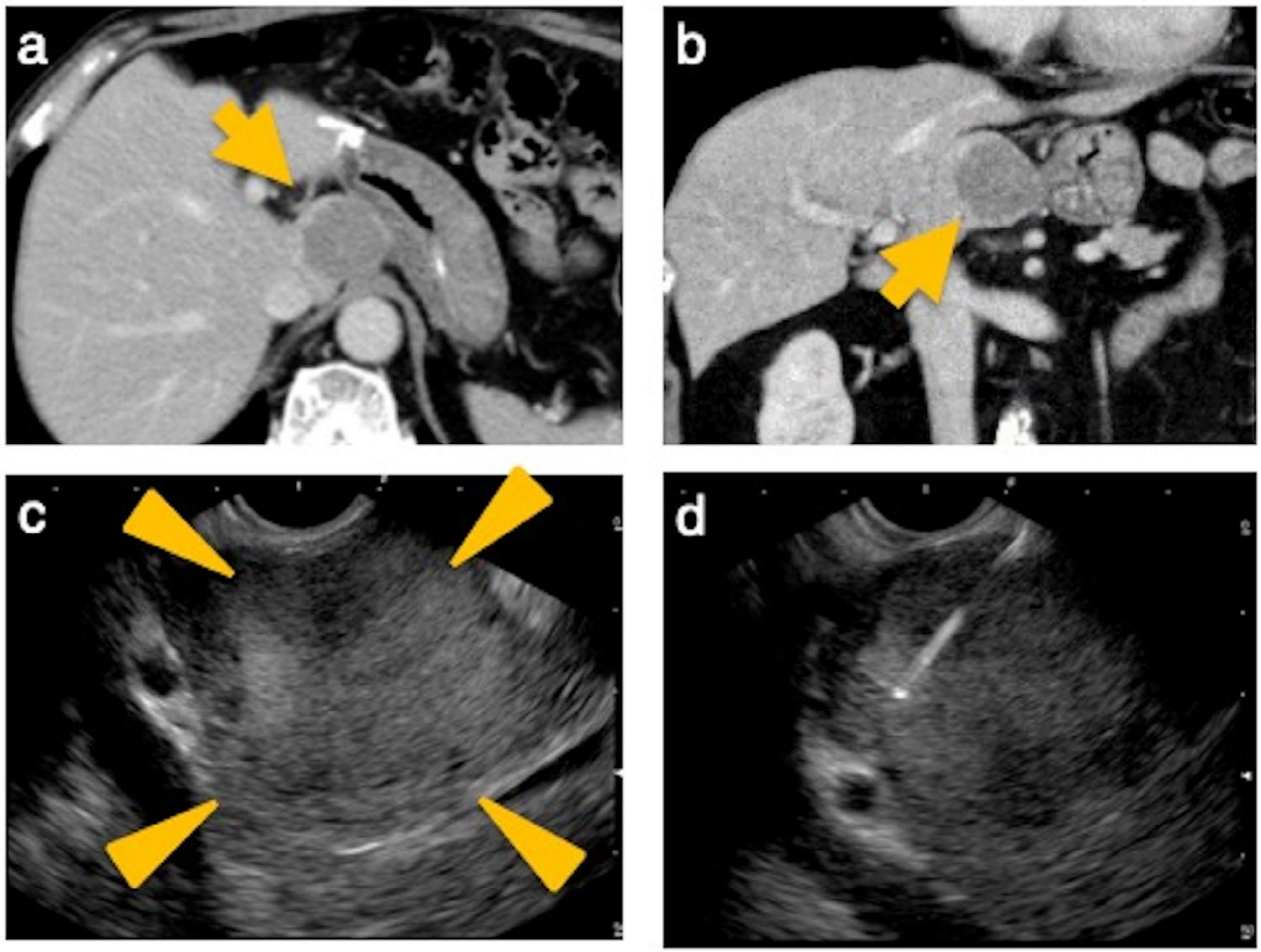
Endoscopic ultrasound‐guided tissue acquisition (EUS‐TA) for a caudate lobe mass. (a) Computed tomography (CT) image (axial). A 30‐mm low‐attenuation mass is observed in the caudate lobe. (b) CT image (coronal). The lesion is located adjacent to the stomach. (c) Endoscopic ultrasound (EUS) image. The lesion was easily visualized. (d) EUS‐TA was performed. The lesion was diagnosed as a metastatic liver tumor.
